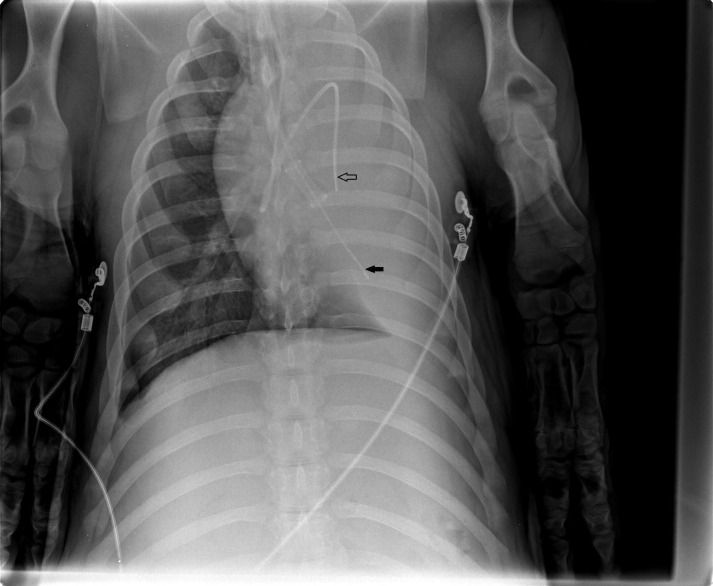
Chest X-ray after one-lung flooding of the left lung, showing the pulmonary artery catheter in the left main artery (arrow) and the intrapulmonary catheter via the left bronchial lumen of the DLT (fat arrow).
